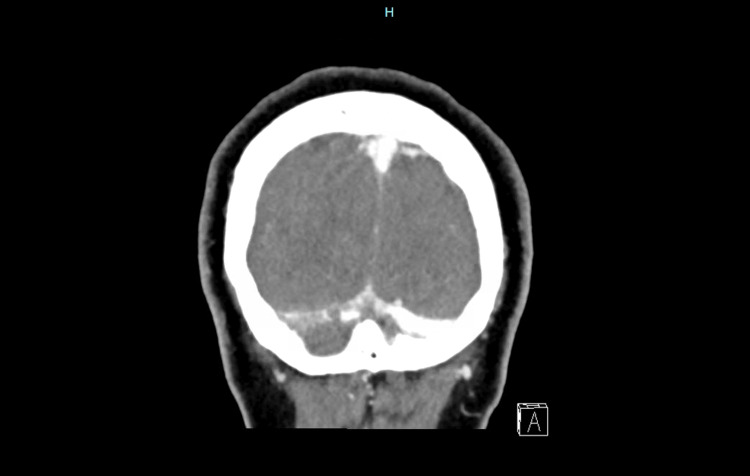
CT venogram showing right transverse sinus thrombosis
